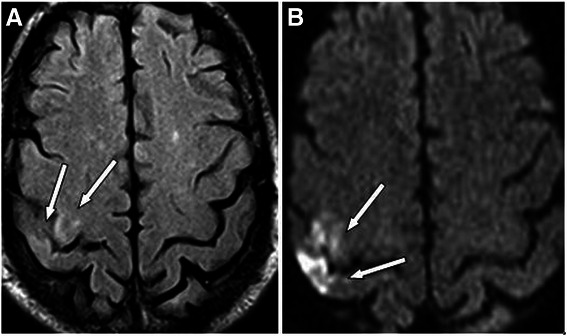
Sixty-eight-year old man with 80 % stenosis of right ICA. Preoperative DWI image shows right occipital chronic ischemia (a). Postoperative DWI image demonstrate new right parietooccipital ischemic lesion (b) (White arrows)
